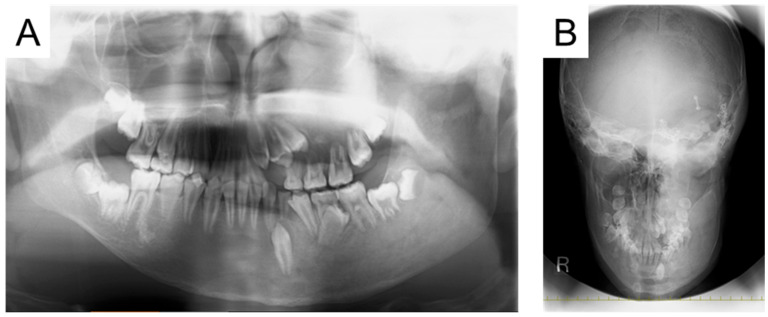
Radiographic examination at the age of 12 years and 9 months ((A): Panoramic photograph, (B): Antero-posterior-view skull).
